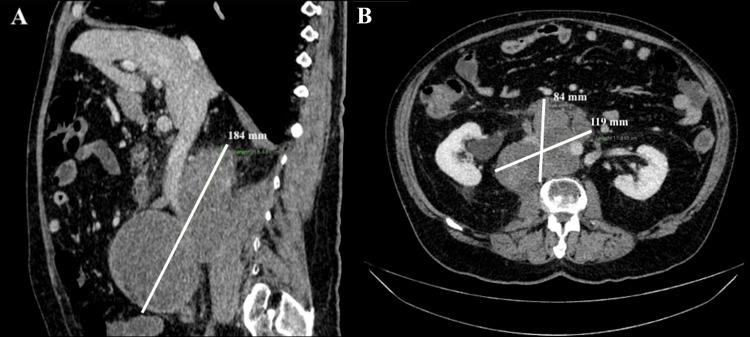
Abdominal and pelvic CT at eight months in sagittal (A) and axial (B) planes The images show a greater decrease in the size of the retroperitoneal lesion eight months after the application of the Lattice radiotherapy technique, with current dimensions 184 × 119 × 82 mm in the longitudinal (A), transverse, and anteroposterior axes (B and C), respectively (delineated within the white lines) CT: computed tomography
